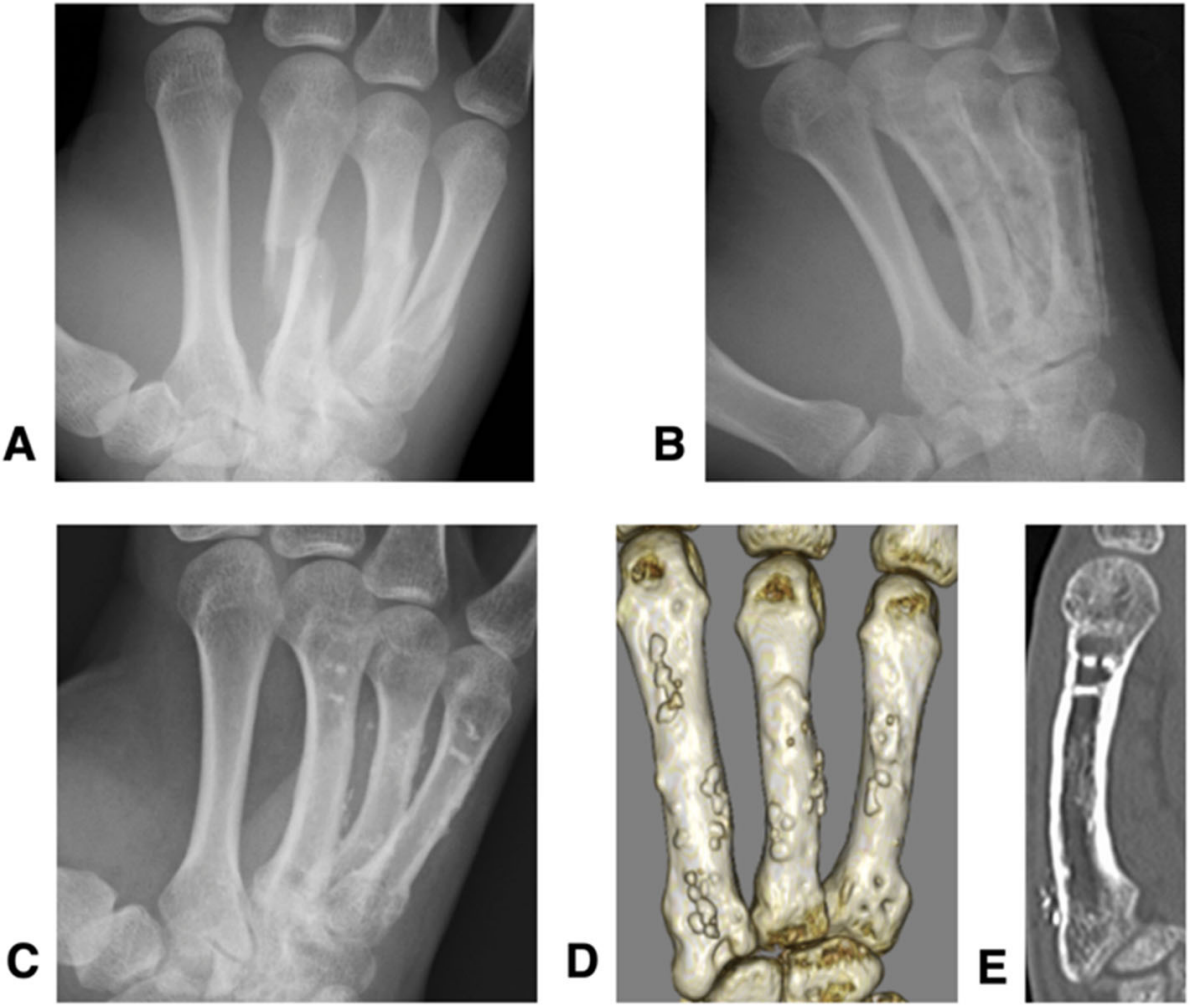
Radiographic images from a 20-year-old male with a comminuted metacarpal fracture of the left middle finger and oblique metacarpal fractures of the left ring and little fingers (Case 6). The a preoperative radiograph, b postoperative radiograph, c final follow-up radiograph (taken 7 years 2 months post-surgery), d final follow-up 3D-computed tomography and e final follow-up plain CT images are shown
